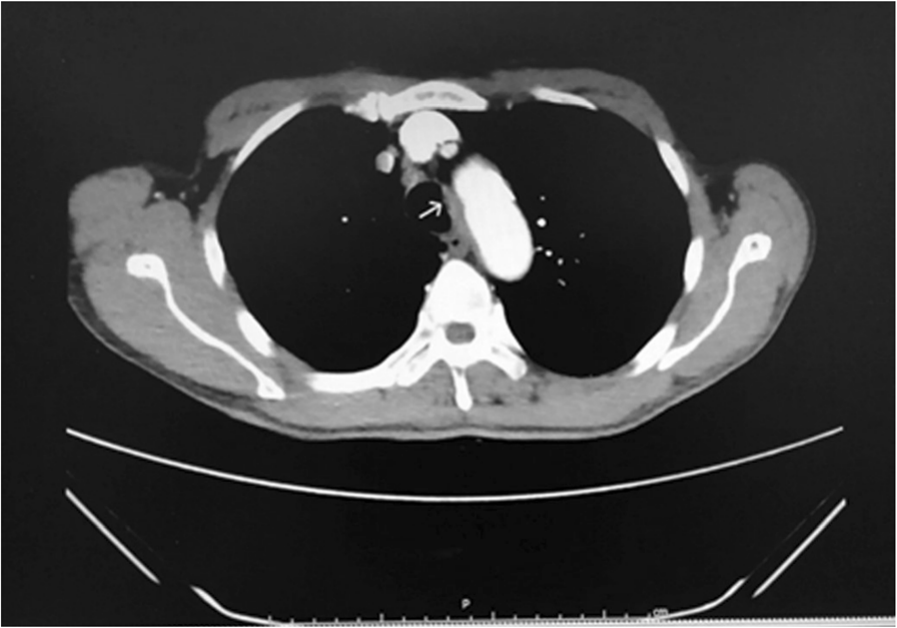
Chest computed tomography (CT) revealed a local thickening in the middle trachea
